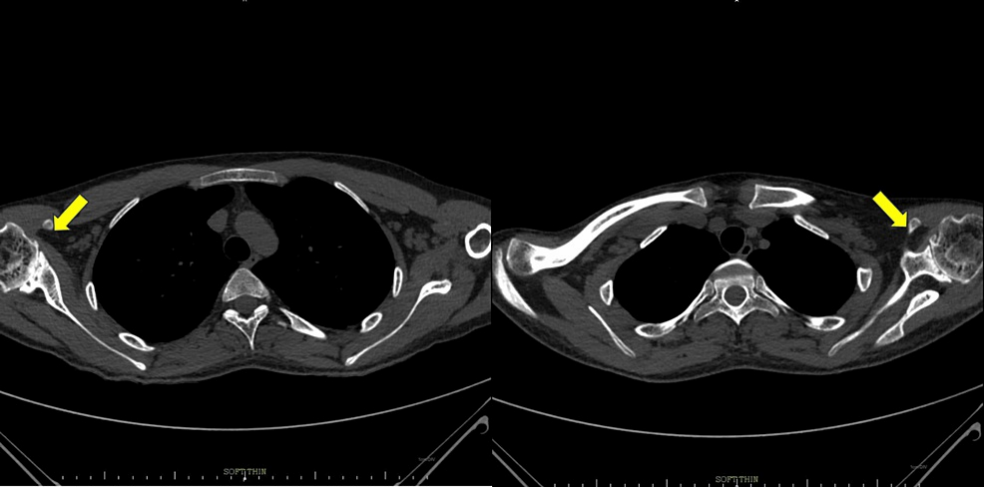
HRCT thorax: axial section No pleuro-parenchymal abnormality was noted. Both the yellow arrows depict bilateral glenohumeral joints showing degenerative changes in the form of loss of humeral head contour, joint narrowing, marginal osteophytes, subchondral sclerosis, and marked osteopenia. HRCT thorax: high-resolution computed tomography of the thorax
